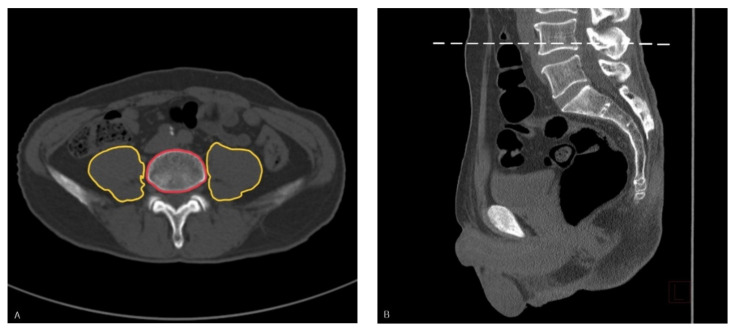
(A) Representative cross-sectional image. Measurement of the right and left psoas muscle areas (outlined in yellow) and vertebral body area (outlined in red) at the L4 level using abdominopelvic computed tomography images; (B) Representative sagittal image. The cross-sectional image was obtained at the level of the dashed line.
